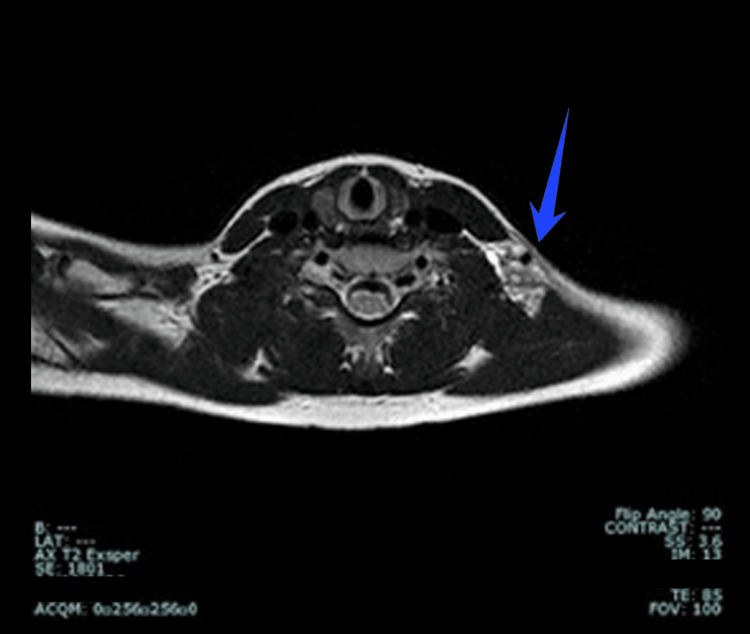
Axial T2-weighted MRI image of the neck Axial T2-weighted image of the neck showing an oval-shaped multiloculated cystic lesion (marked by arrow) in the left posterior triangle of the neck showing multiple fluid-debris levels without surrounding inflammatory changes.
